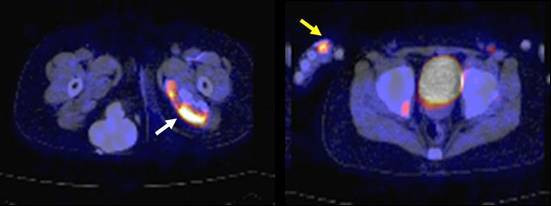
Findings of 18 FDG-PET/CT showing "inflammatory calcinosis"in August 2006. Subcutaneous and intramuscular calcification were recognized as in conventional plain CT. Hot spots (white arrow) along with intramuscular calcification indicate inflammation. Even the small lesion of inflammation around a right digit (yellow arrow) can be seen clearly.
